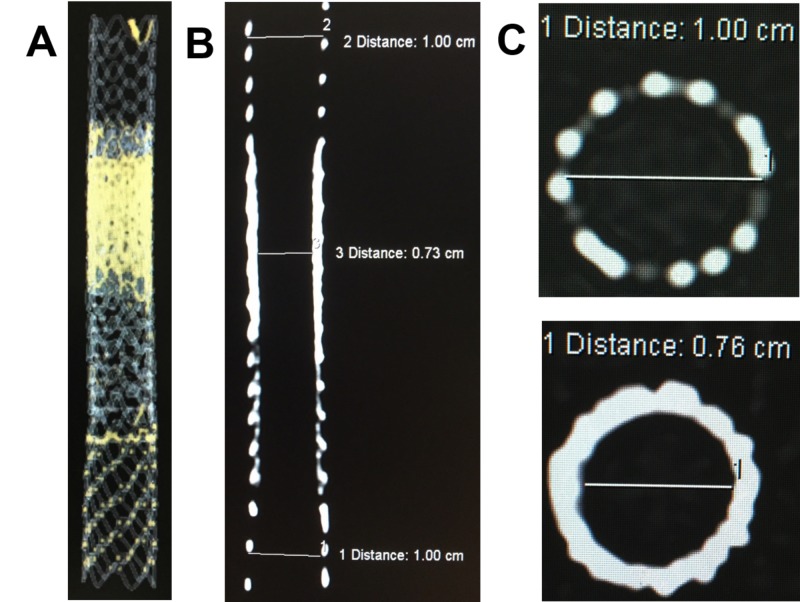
A) 3D CT image of the Viatorr and Viabahn stents laminated. B) Coronal CT image showing the smooth hourglass tapering from the onion skin technique. C) Shows the axial CT image of a 10 mm Viatorr stent (top) and axial CT image through onion skin layers of Viabahn stent grafts with a narrowing of the lumen to 0.76 mm (bottom). CT: computed tomography; Viabahn: WL Gore and Associates, Flagstaff, AZ, USA; Viatorr: WL Gore and Associates, Flagstaff, AZ, USA
